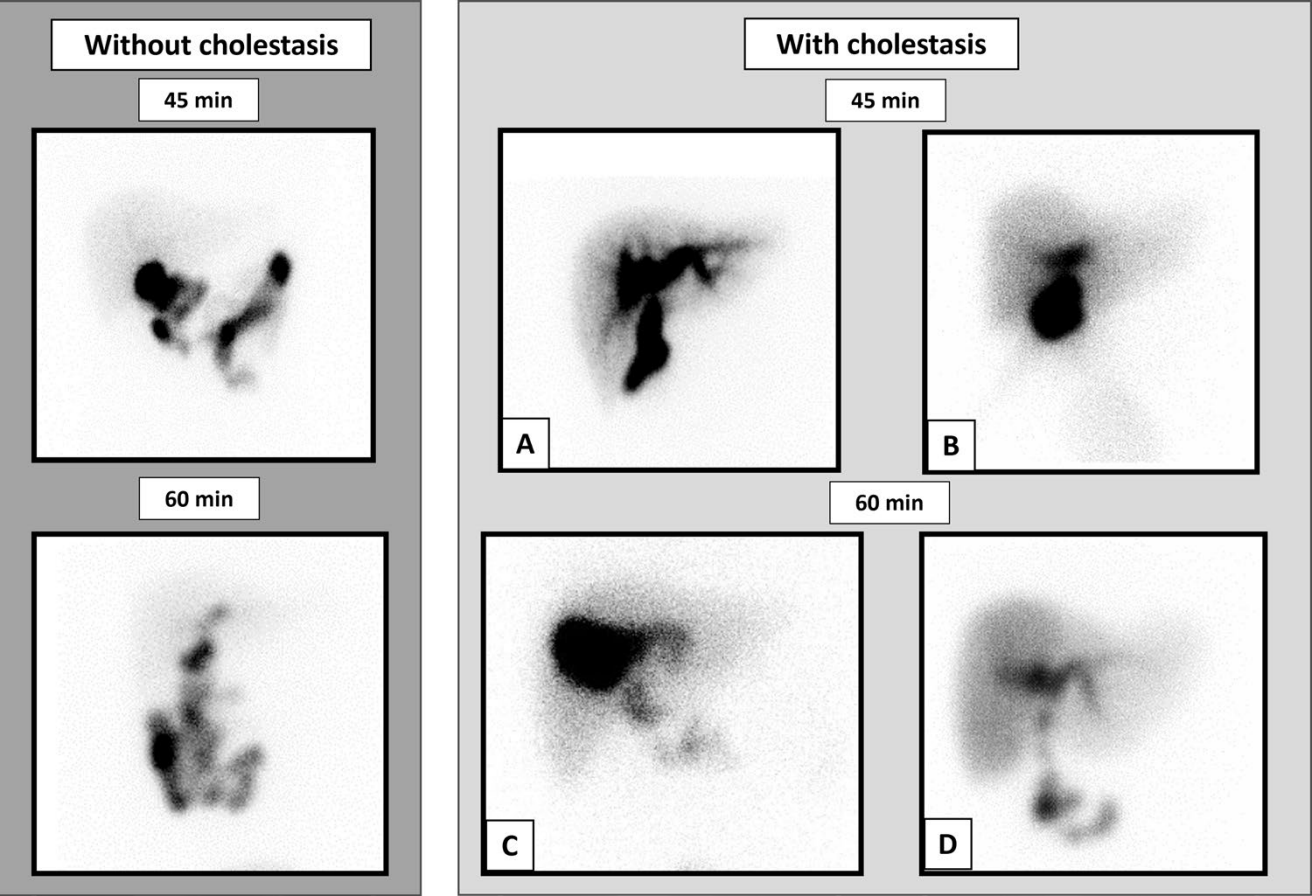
Typical images of cholestasis and non-cholestasis on ^99m^Tc-N-pyridoxyl-5-methyltryptophan hepatobiliary scintigraphy. With cholestasis: Upper row: Nuclide excretion is not observed beyond the Roux-en-Y anastomosis into the intestinal tract at 45 min after injection. A: Dilatation of the intrahepatic bile ducts and no nuclide excretion beyond the Roux-en-Y anastomosis. B: Dilatation of the intrahepatic bile ducts and Roux-en-Y anastomosis. Lower row: Nuclide remains within the liver; this is clearly delineated at 60 min after injection. C: Nuclide remains in the intrahepatic bile ducts and liver. D: Nuclide is retained within the right side of the liver
